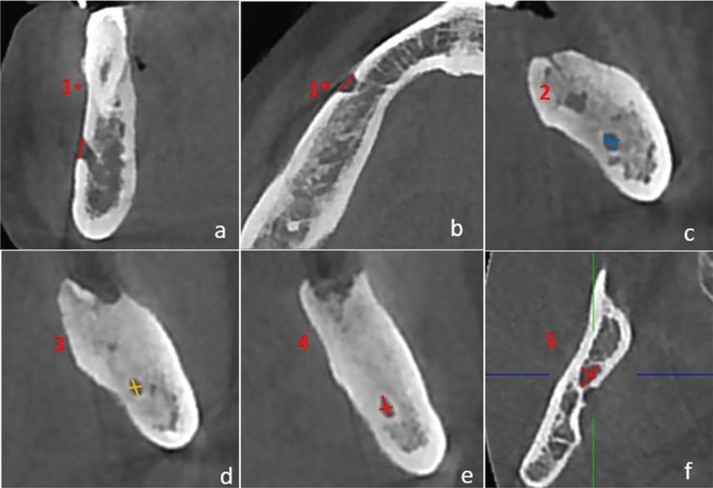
The demonstration of measurements on CBCT images. a. section 1+; cross-sectional measurements of mental foramen, b. section 1*; axial measurements of mental foramen. c-e. The minimum and maximum diametric measurements through mandibular canal on section 2-4. f. The minimum and maximum diametric measurements of mandibular foramen on section 5.
